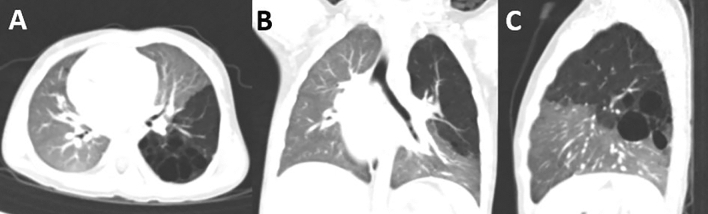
Congenital pulmonary airway malformation involving multiple lobes on a chest computed tomography scan: the apicoposterior segment, anterior segment (S1-3) of the left upper lobe and superior segment (S6) of the left lower lobe. (A) axial view (B) coronal view (C) sagittal view.
